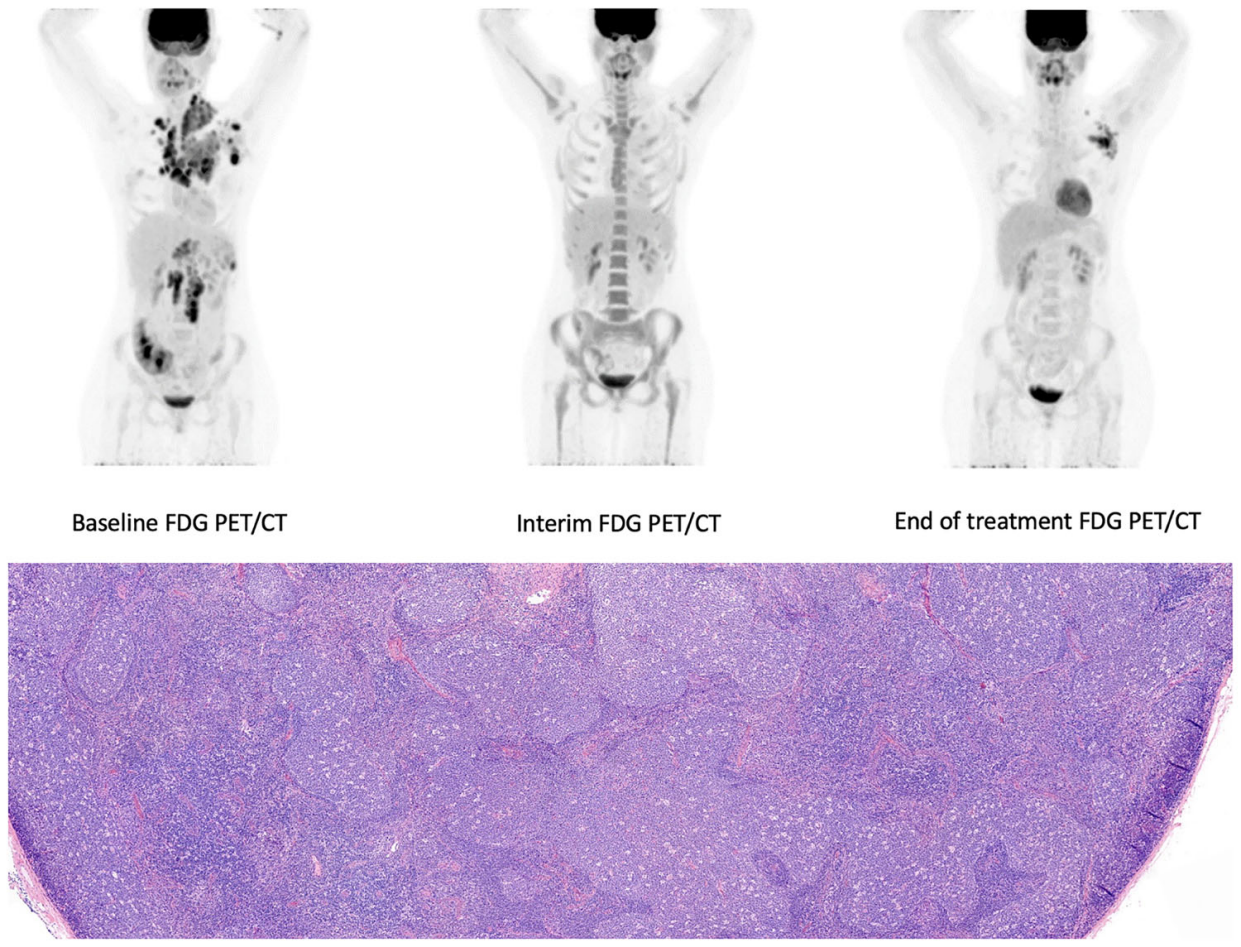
A 21‐year‐old woman presented with nodular sclerosis Hodgkin lymphoma stage IIIA, IPS 2 with a baseline ^18^F‐fluorodeoxyglucose positron emission tomography and computed tomography (FDG PET/CT) showing initial involved lymph nodes in both sides of the neck, left axilla, mediastinum, hili, para‐aortic, left iliac, and involvement of the spleen. After one course of BrAVD (brentuximab‐vedotin, adriamycine, vinblastine, dacarbazine), the interim FDG PET/CT scan was negative, classified as a complete metabolic response. A third FDG PET/CT scan was performed after completion of her chemotherapy consisting of six courses of BrAVD. This scan showed a reappearance of FDG avid lymph nodes, with a Deauville score of 5, in the original sites in the left axilla and neck. The most FDG avid lymph node was excised and revealed florid follicular hyperplasia instead of recurrence of Hodgkin lymphoma.
